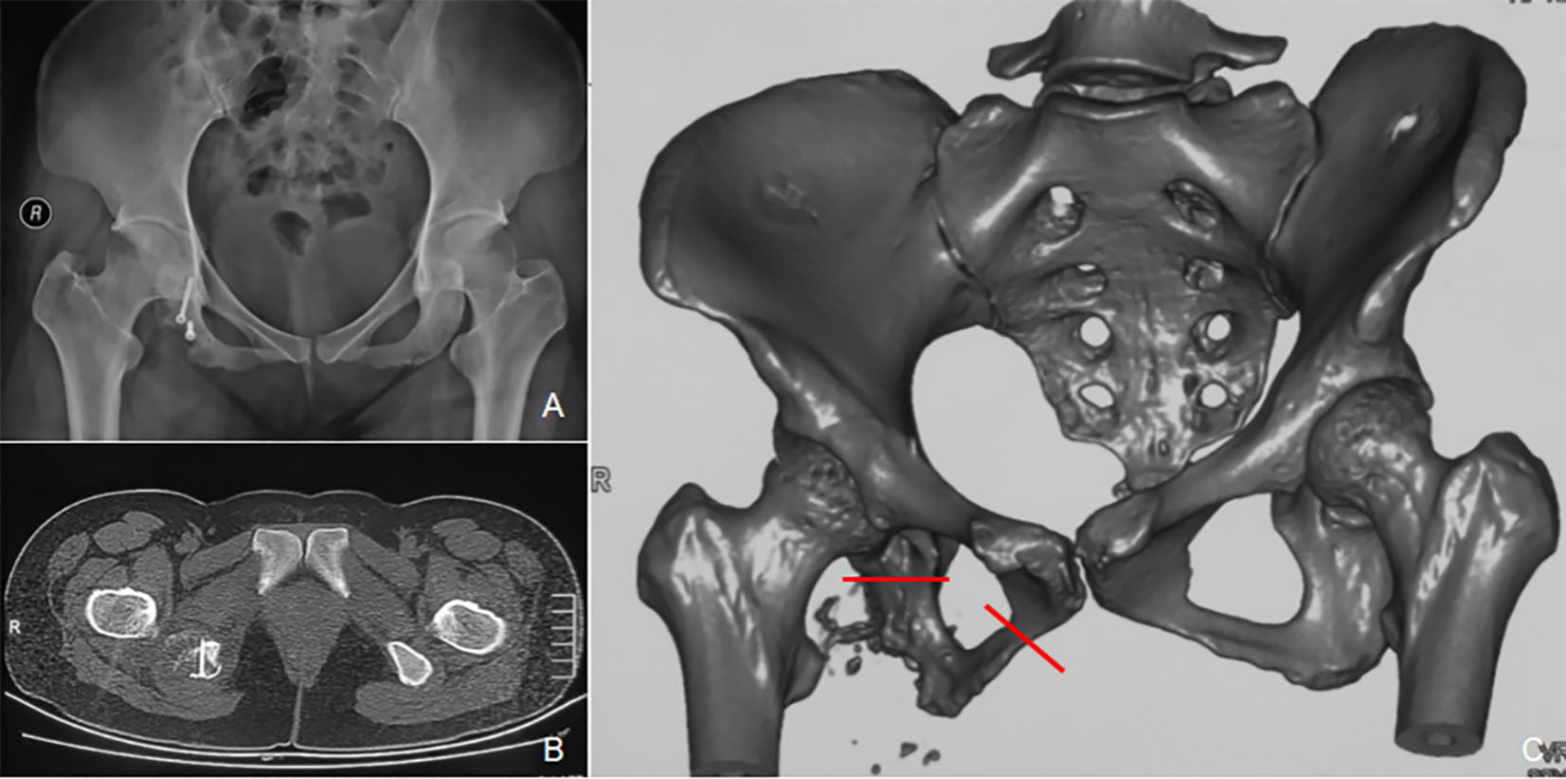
A 31-year-old female patient with a recurrence of right ischiatic tuberous chondrosarcoma after curettage. (A) Pelvic radiographs displaying that internal fixation was in place and that the right ischiatic tubercle was lacking in the bone and soft tissue mass around it. (B) CT scans revealed lytic bone destruction of the right ischiatic tubercle with soft tissue mass and punctate calcification. (C) Preoperative plan: An extended resection of the right ischiatic tubercle was proposed (the red line indicates the intraoperative osteotomy line drawn up before surgery).
